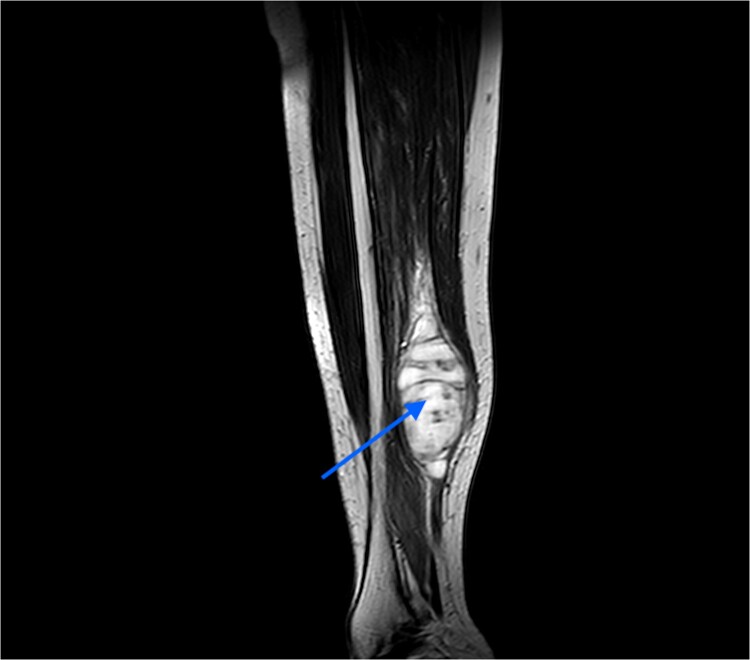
MRI image taken in coronal view, which was suggestive of a spindle-shaped mass lesion (indicated by arrow) located in the posterior compartment of the leg.
